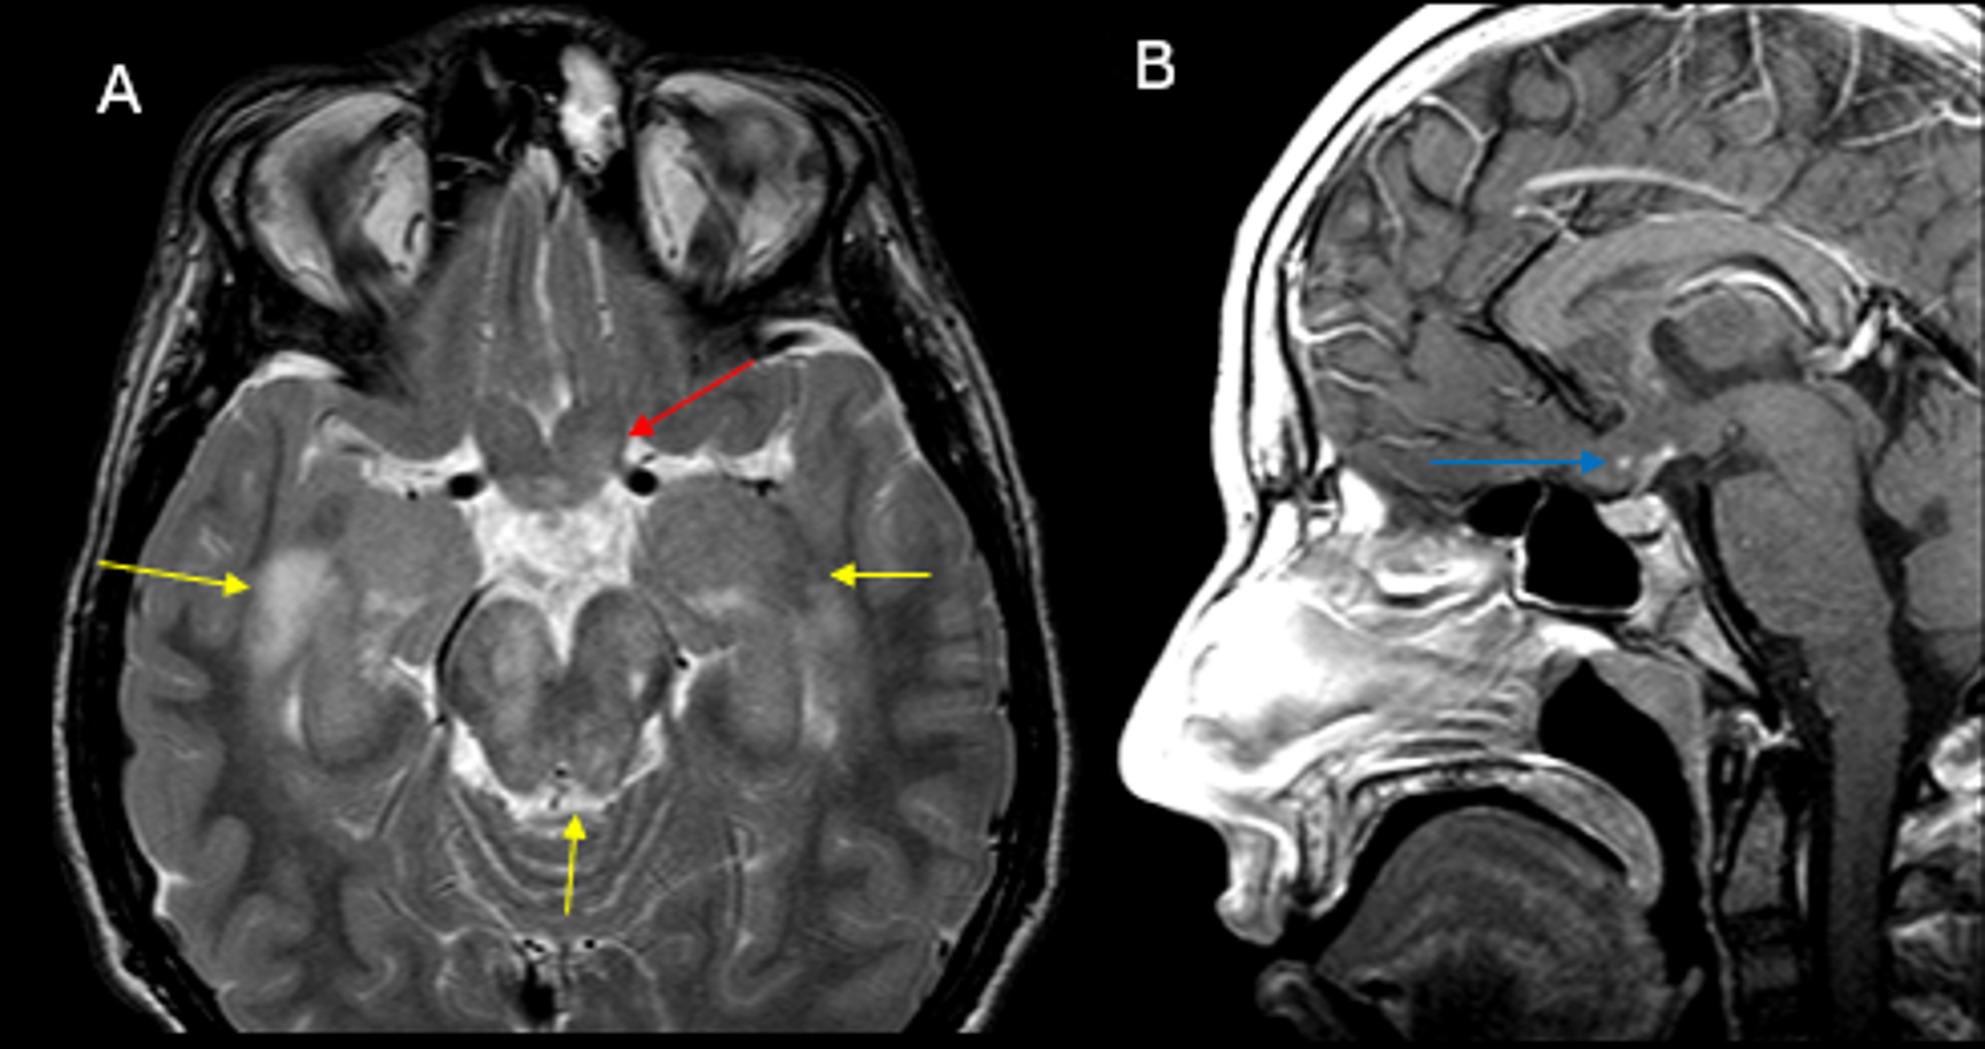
(A) Axial T2-weighted image of the orbits shows abnormal thickening of optic chiasm and pre-chiasmatic optic nerves with abnormal hyperintense T2 signal (red arrow), consistent with optic pathway glioma in this NF1 patient. Multifocal areas of spongiform myelin with hyperintense T2 signal are present in the bilateral medial temporal lobes and brainstem (yellow arrows). (B) Sagittal T1-weighted image of the sella redemonstrates the optic pathway glioma, showing minimal enhancement in the chiasmatic component (blue arrow in B)
